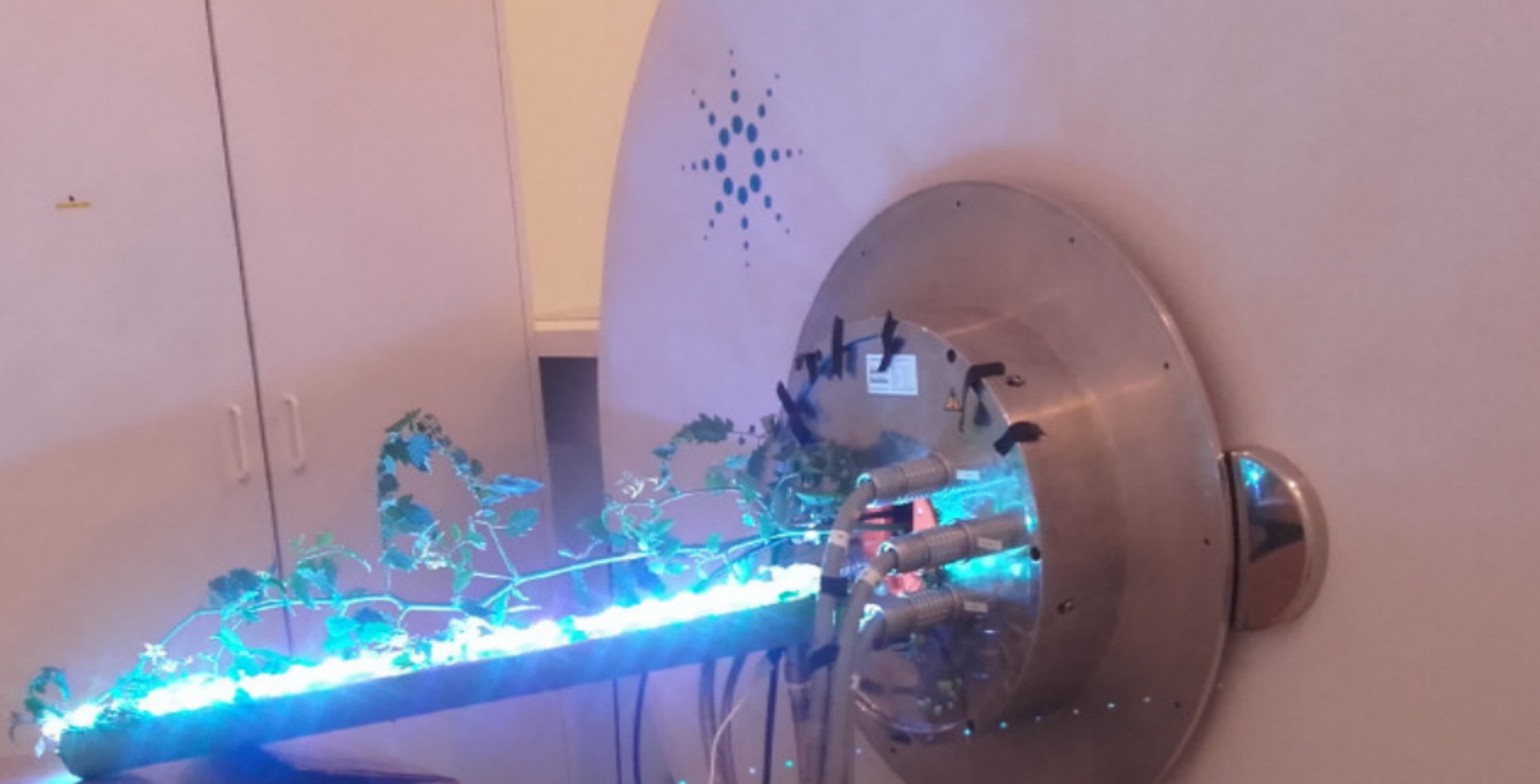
Tomato plant installation. The tomato plant is installed inside the MRI magnet. The external part of the plant is enlightened by the LED panel.
